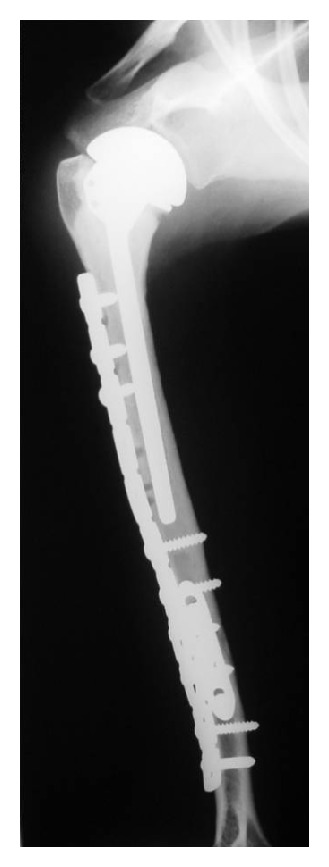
Anteroposterior radiograph of an APC of the proximal humerus showing adequate union of the junction.
